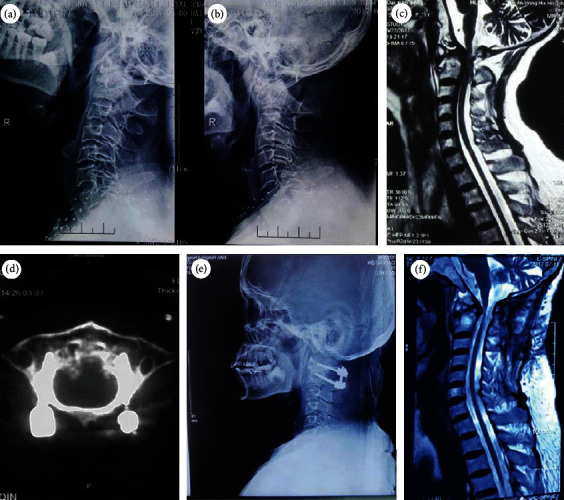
(a, b) Flexion-extension X-rays show atlantoaxial dislocation and reduction; (c) atlantoaxial reduction without spinal cord compression on preoperative dynamic MRI; (d) postoperative CT shows atlas arch conservation; (e) postoperative X-ray shows atlantoaxial reduction; (f) postoperative MRI shows spinal cord decompression.
